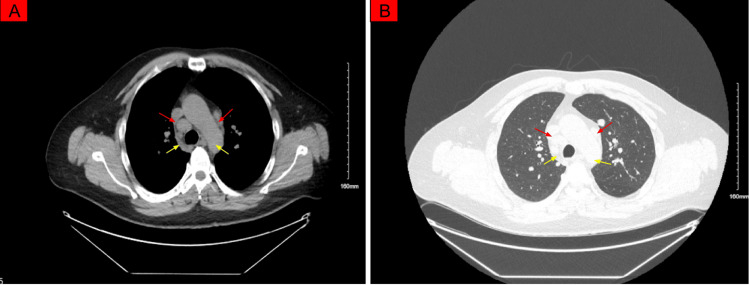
A: Axial CT scan showing bilateral hilar lymphadenopathy (red arrows) and mediastinal nodes (yellow arrows). B: Coronal CT scan highlighting noncalcified pulmonary nodules (red arrows) and hilar lymphadenopathy (yellow arrows).
